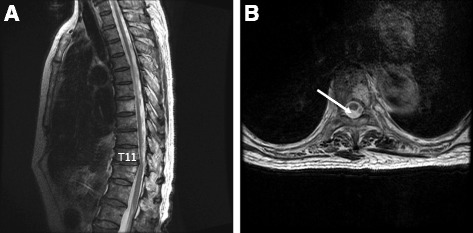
a Sagittal MRI on postoperative day 93 demonstrated that the hyperintense region was localized to T11. b Axial MRI of the T8 spinal cord level. Scarring of the infarction occurred, and the fluid space was extended
